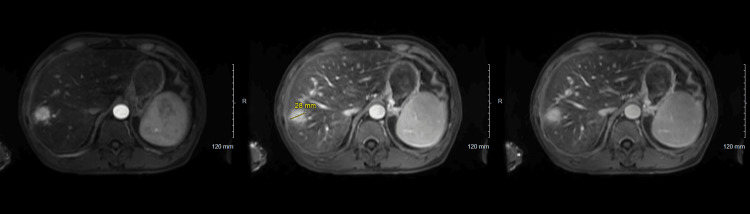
Contrast imaging MRI MRI images demonstrate a mass highly suspicious for hepatocellular carcinoma in the right hepatic lobe. Moving from left to right are the images of three post-contrast phases: arterial phase, portal phase, and vasculo-Kupffer phase.
